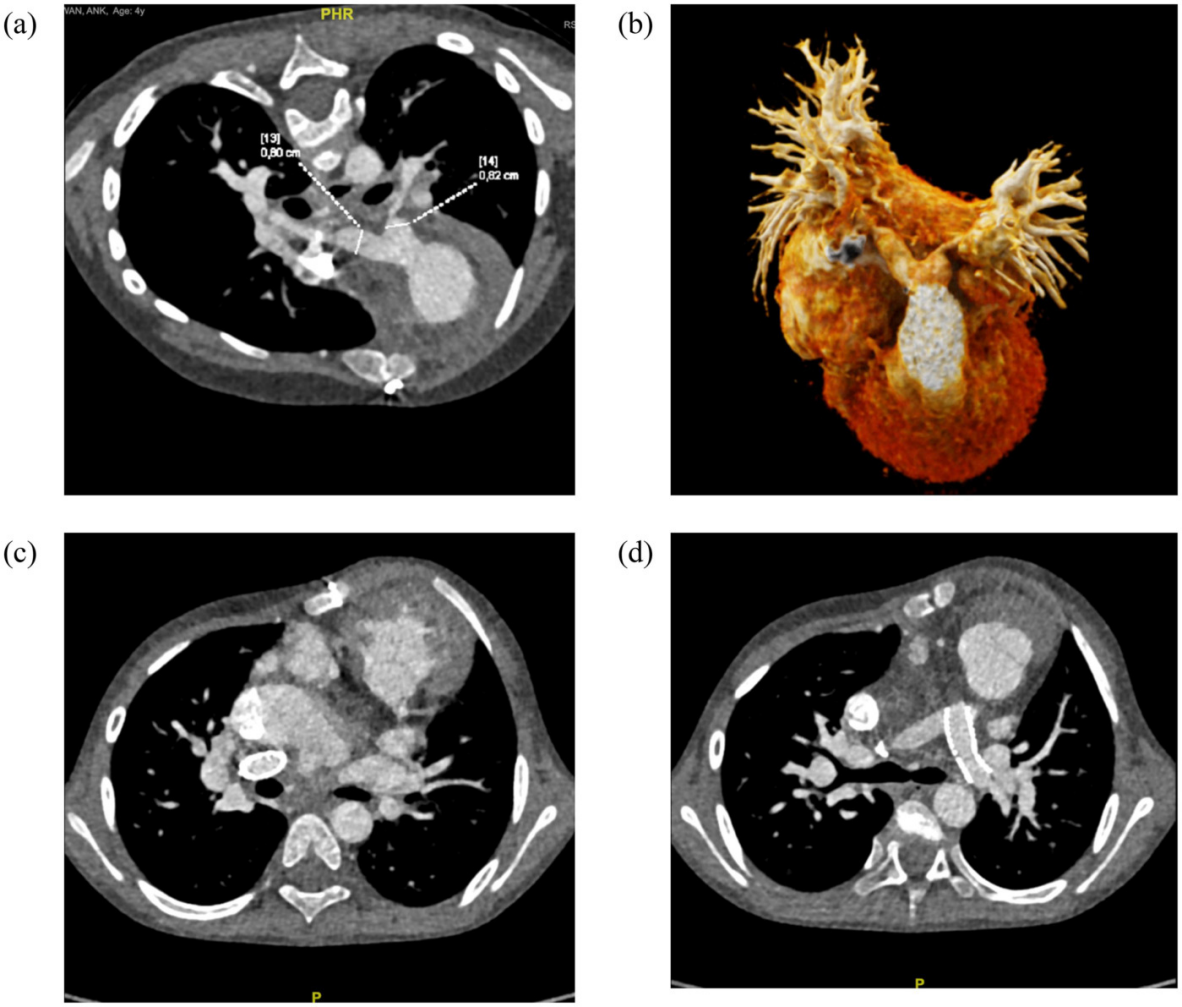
Follow-up CT imaging of a patient who underwent bilateral pulmonary artery (PA) stenting. (a) Baseline measurements of the left (LPA) and right pulmonary artery (RPA) diameters. (b) Three-dimensional reconstruction demonstrating bilateral PA stenosis prior to intervention. (c) Post-stenting appearance of the RPA. (d) Post-stenting appearance of the LPA.
